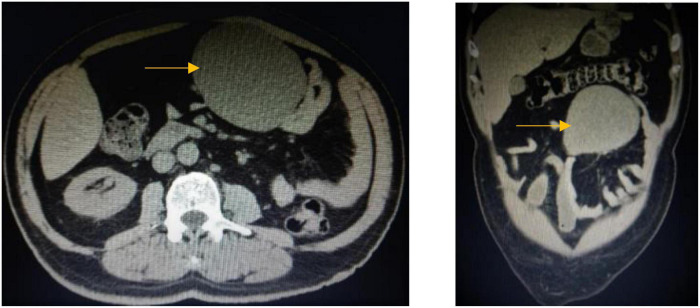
CT scan of the abdomen, coronal, and axial images shows large well-defined opacity at mesentery on the left side of the upper abdomen surrounded by small and large bowel loops with no enlarged mesenteric or retroperitoneal lymph nodes.
